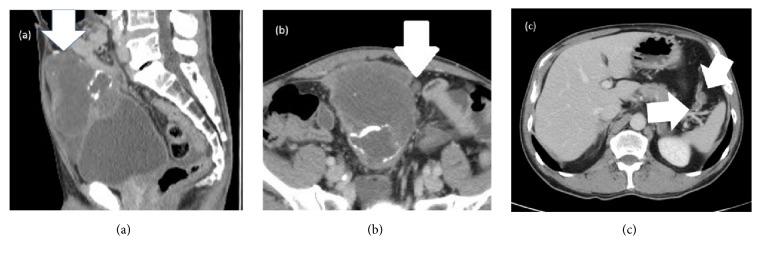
Abdominal computed tomographic image taken before systemic chemotherapy, showing (a) a 9-cm mass extending from the bladder to the umbilicus (white arrow) and ((b) and (c)) intraperitoneal nodules (white arrows).
